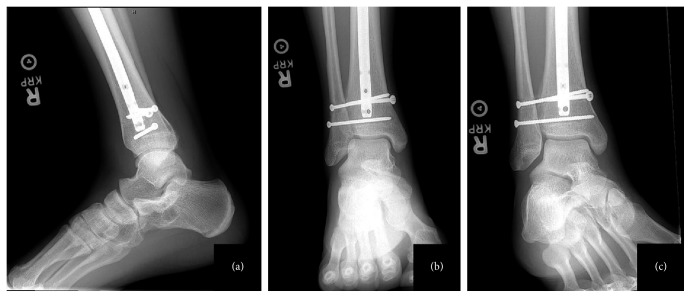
Postoperative lateral (a), AP (b), and mortise (c) radiographs showing 2 quadricortical screws stabilizing the syndesmosis with no evidence of hardware failure.
